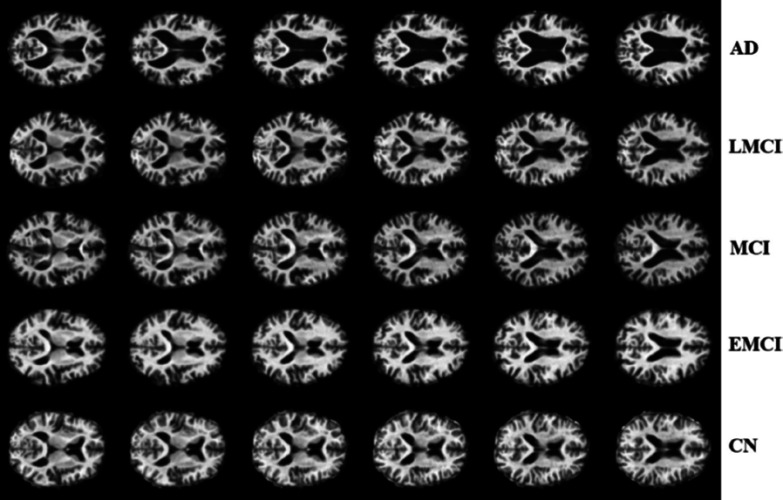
MRI in CN, EMCI, MCI, LMCI, and AD. Figure adapted from [58]
